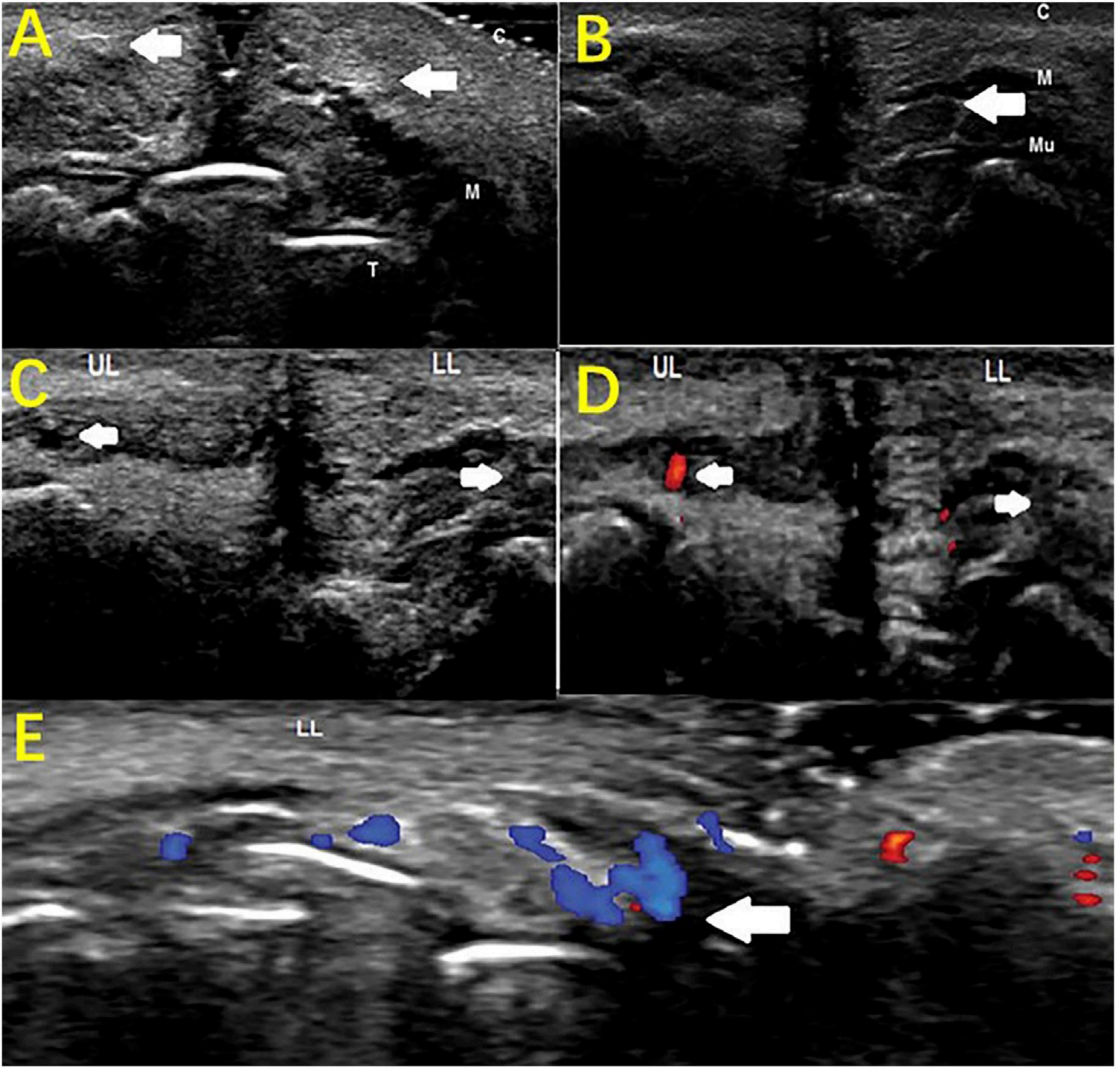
Monitoring complications of esthetic treatments by HFUS imaging. Sagittal plane (A) Hyperechoic lips (arrows); (B) Hypoechogenic area (filler deposit, arrow) surrounded by a hyperechoic region (T-teeth, M-orbicularis muscle, C-cutaneous tissue, Mu-mucosa). Sagittal plane (C) B-mode sonogram: The superior labial artery (left arrow), invisible inferior artery (right arrow); (D) Color Doppler sonogram: visible blood flow of the superior labial artery (left arrow), invisible blood flow of the inferior labial artery (right arrow); (LL: lower lip; UL: upper lip). Transverse plane (E) Color Doppler sonogram: collateral circulation of the left inferior labial artery (arrow).
